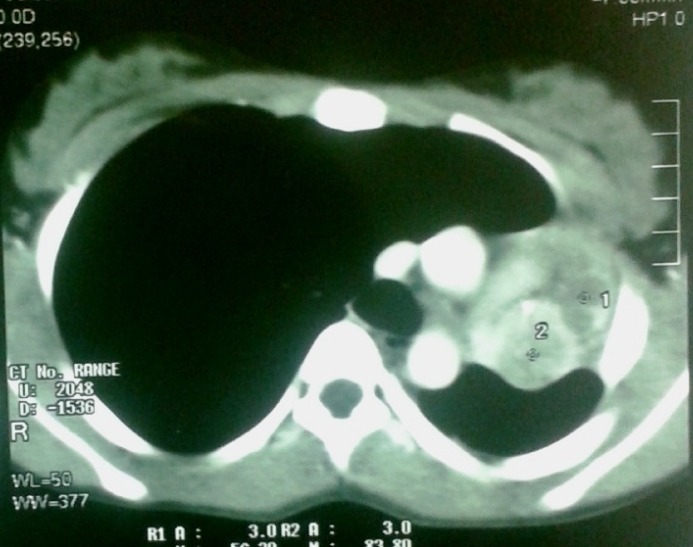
Figure 1:CT scan of chest showing obstruction of left main bronchus with resultant collapse of left lung and mediastinal shift.
